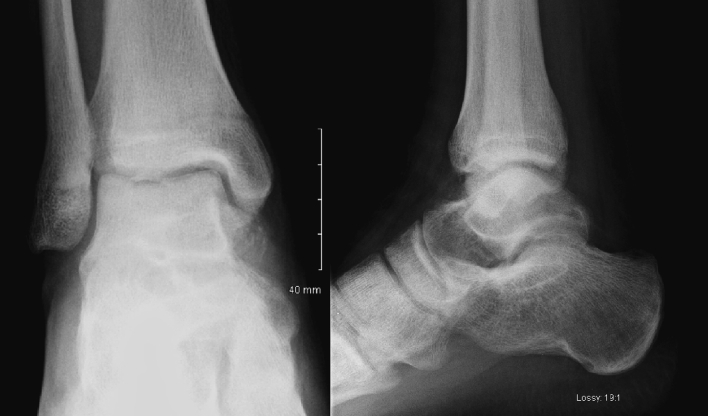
(A, B): Post-operative AP and Lateral Ankle radiographs at 2 weeks. There was a heterogeneous appearance within the anterior process of the talus, in keeping with the recent surgical excision. There was interval development of marked osteopenia, particularly within the mid and hindfoot. There was also a further ill-defined periosteal reaction surrounding the bones of the midfoot.
